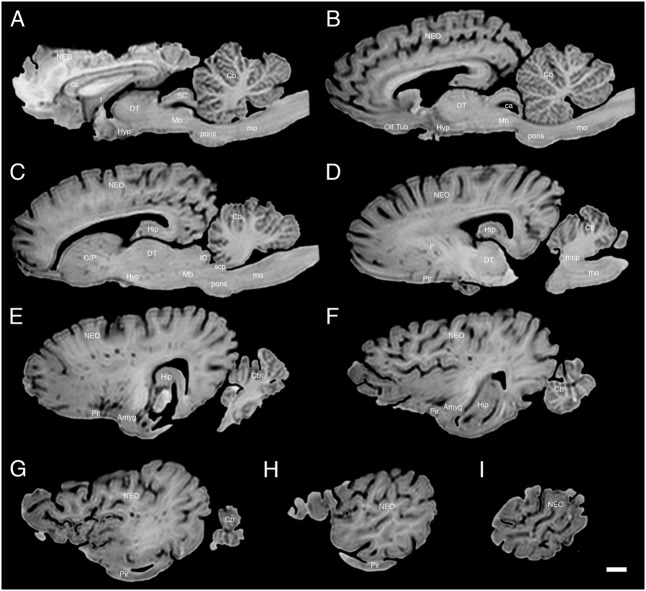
A series of sagittal structural MR images through the brain of the white rhinoceros (*C. simum*). (A) is the most medial section and (I) is the most lateral section, with each section having a thickness of 2 mm and each section being 6 mm apart. Note the typically mammalian topography of the various regions and structures of the brain. Scale bar = 2 cm. Amyg, amygdaloid body; C, caudate nucleus; ca, cerebral aqueduct; Cb, cerebellum; cc, corpus callosum; DT, dorsal thalamus; f, fornix; Hip, hippocampus; Hyp, hypothalamus; IC, inferior colliculus; NEO, neocortex; Mb, midbrain; mcp, middle cerebellar peduncle; mo, medulla oblongata; Olf. Tub, olfactory tubercle; P, putamen; Pir, piriform cortex; SC, superior colliculus; scp, superior cerebellar peduncle.
